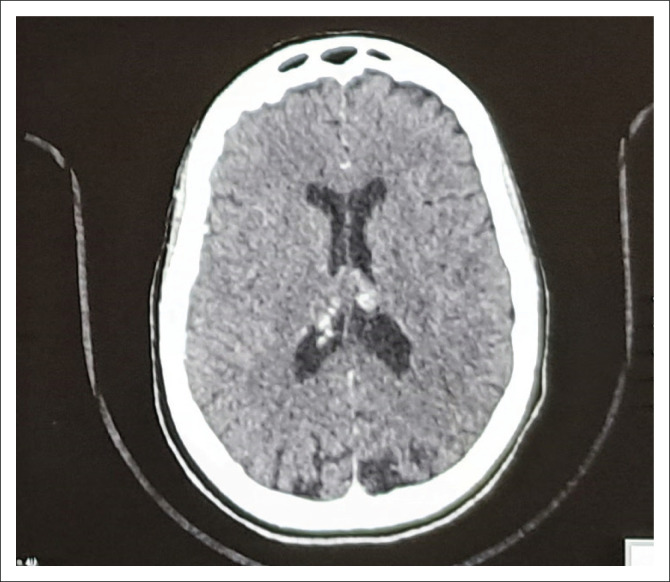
A post-contrast computed tomography scan of the brain showing brain atrophy most prominent posteriorly, in keeping with this diagnosis.
